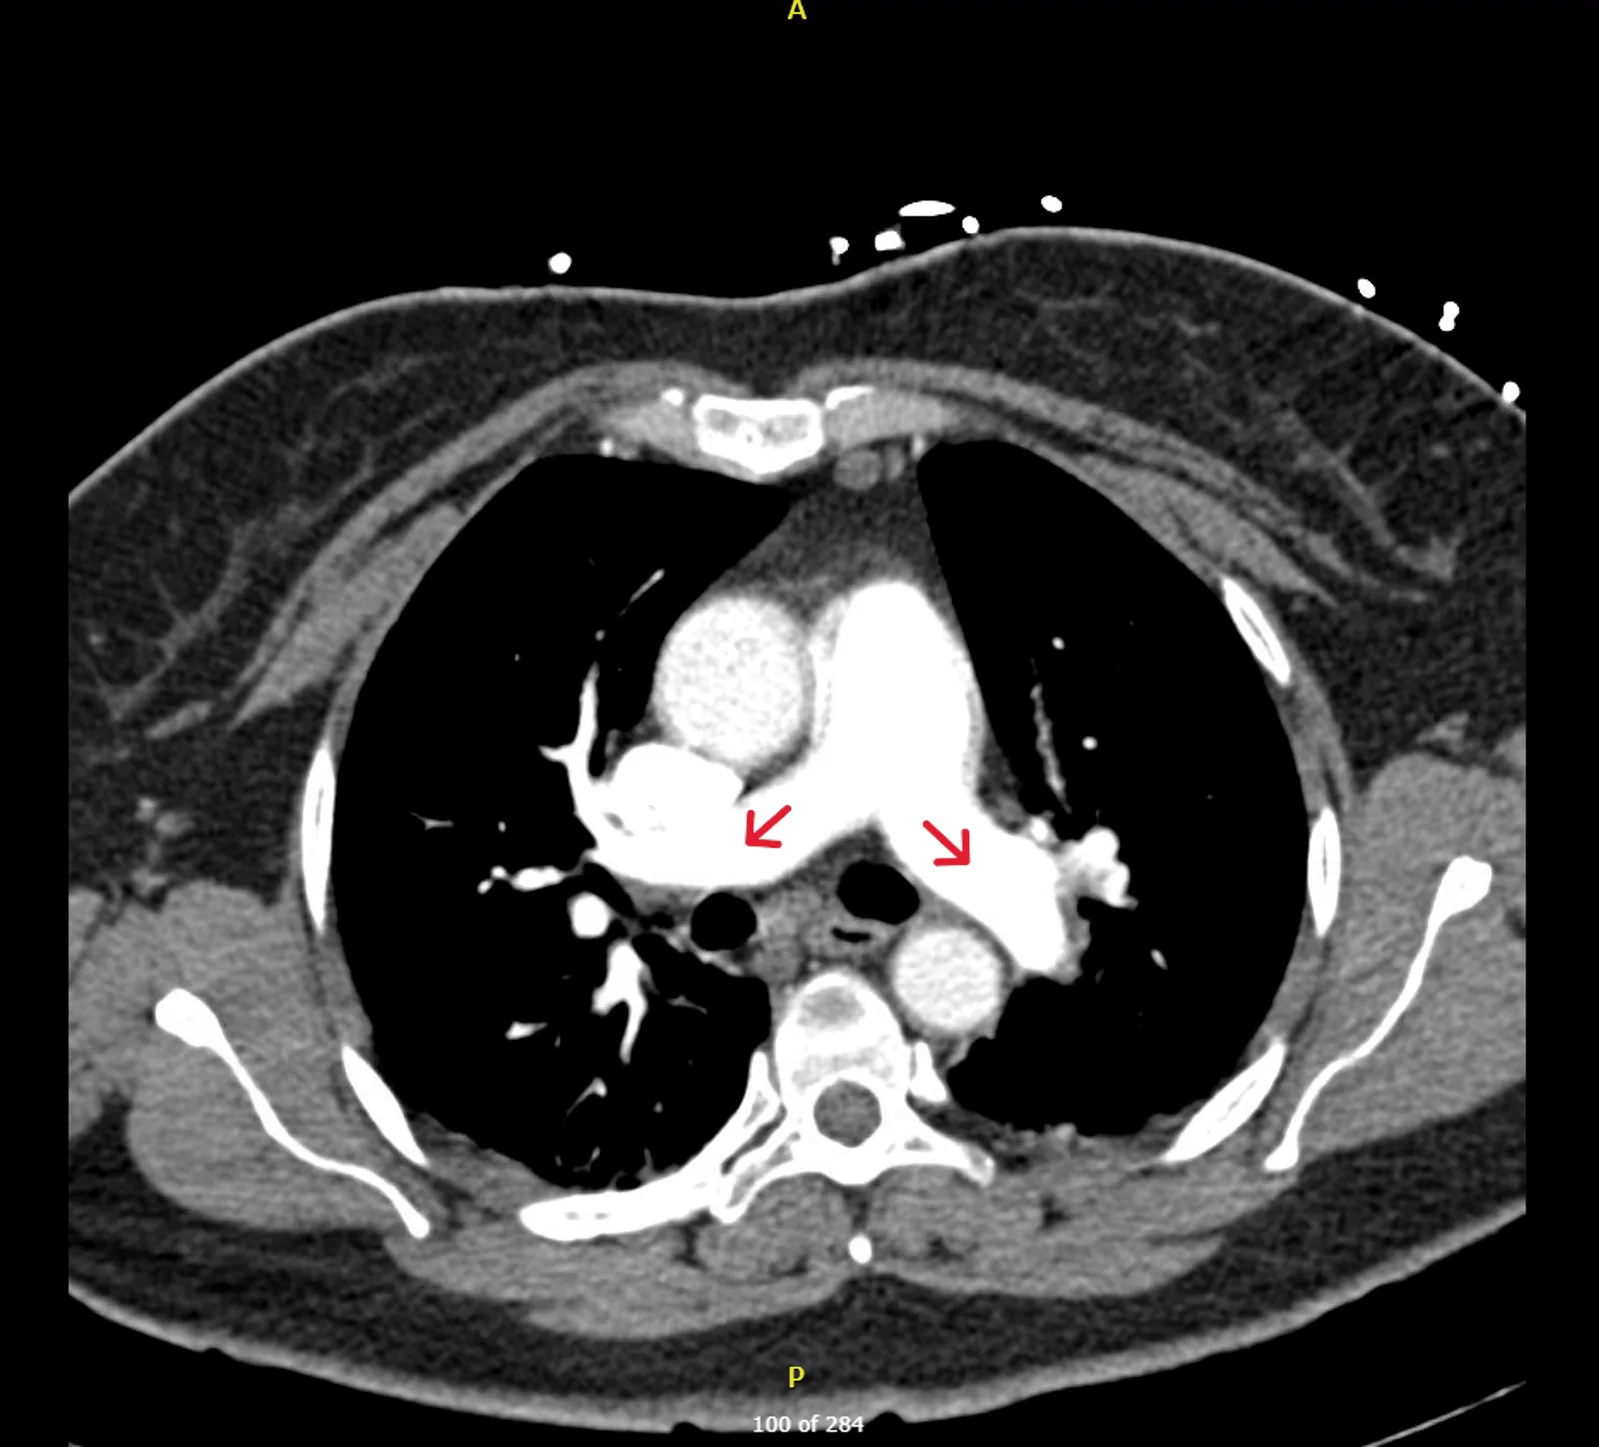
Computed tomography pulmonary angiography demonstrating no evidence of acute pulmonary embolism. Representative axial slice obtained during the patient's second emergency department presentation. Red arrows indicate the contrast-opacified right and left main pulmonary arteries without intraluminal filling defects.
